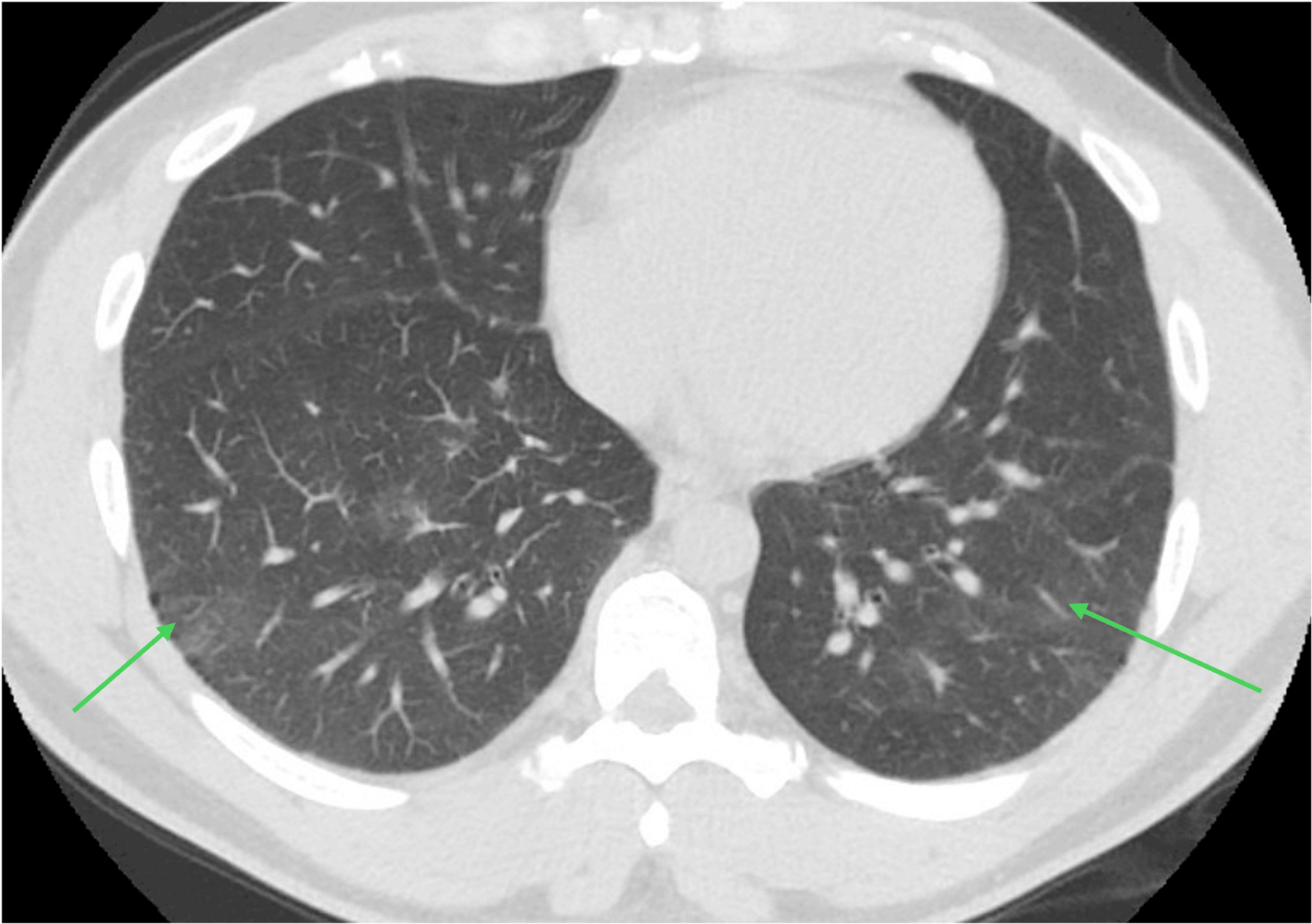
CT chest taken in August 2021, three months after the patient stopped using the CPAP machine, showed an interval decrease with mild residual GGO CPAP, continuous positive airway pressure; GGO, ground-glass opacities.
